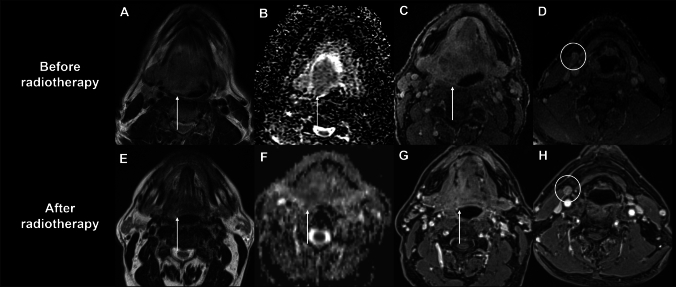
An example of low agreement in the evaluation of an oropharyngeal squamous cell carcinoma (OPSCC) of the base of the tongue on the first post-radiotherapy magnetic resonance imaging. A and E. T2-weighted images; B and F. Apparent diffusion coefficient maps; C, D, G and H. T1-weighted images after contrast agent administration. A, B, C and D show a large OPSCC (arrows) with enlarged cervical lymph nodes (circle) before treatment. E, F, G and H show partial resolution of OPSCC with persistent enlarged cervical lymph node (circle) after treatment. There was variable disagreement among all readers in classifying the individual NI-RADS features (lymph node status, primary tumor size, and primary site signal on T2-weighted, contrast-enhanced, and diffusion-weighted images). The final NI-RADS category assigned was 1 for the general radiologist, 2 for the expert radiologists and a radiology resident, and 3 for a radiology resident
